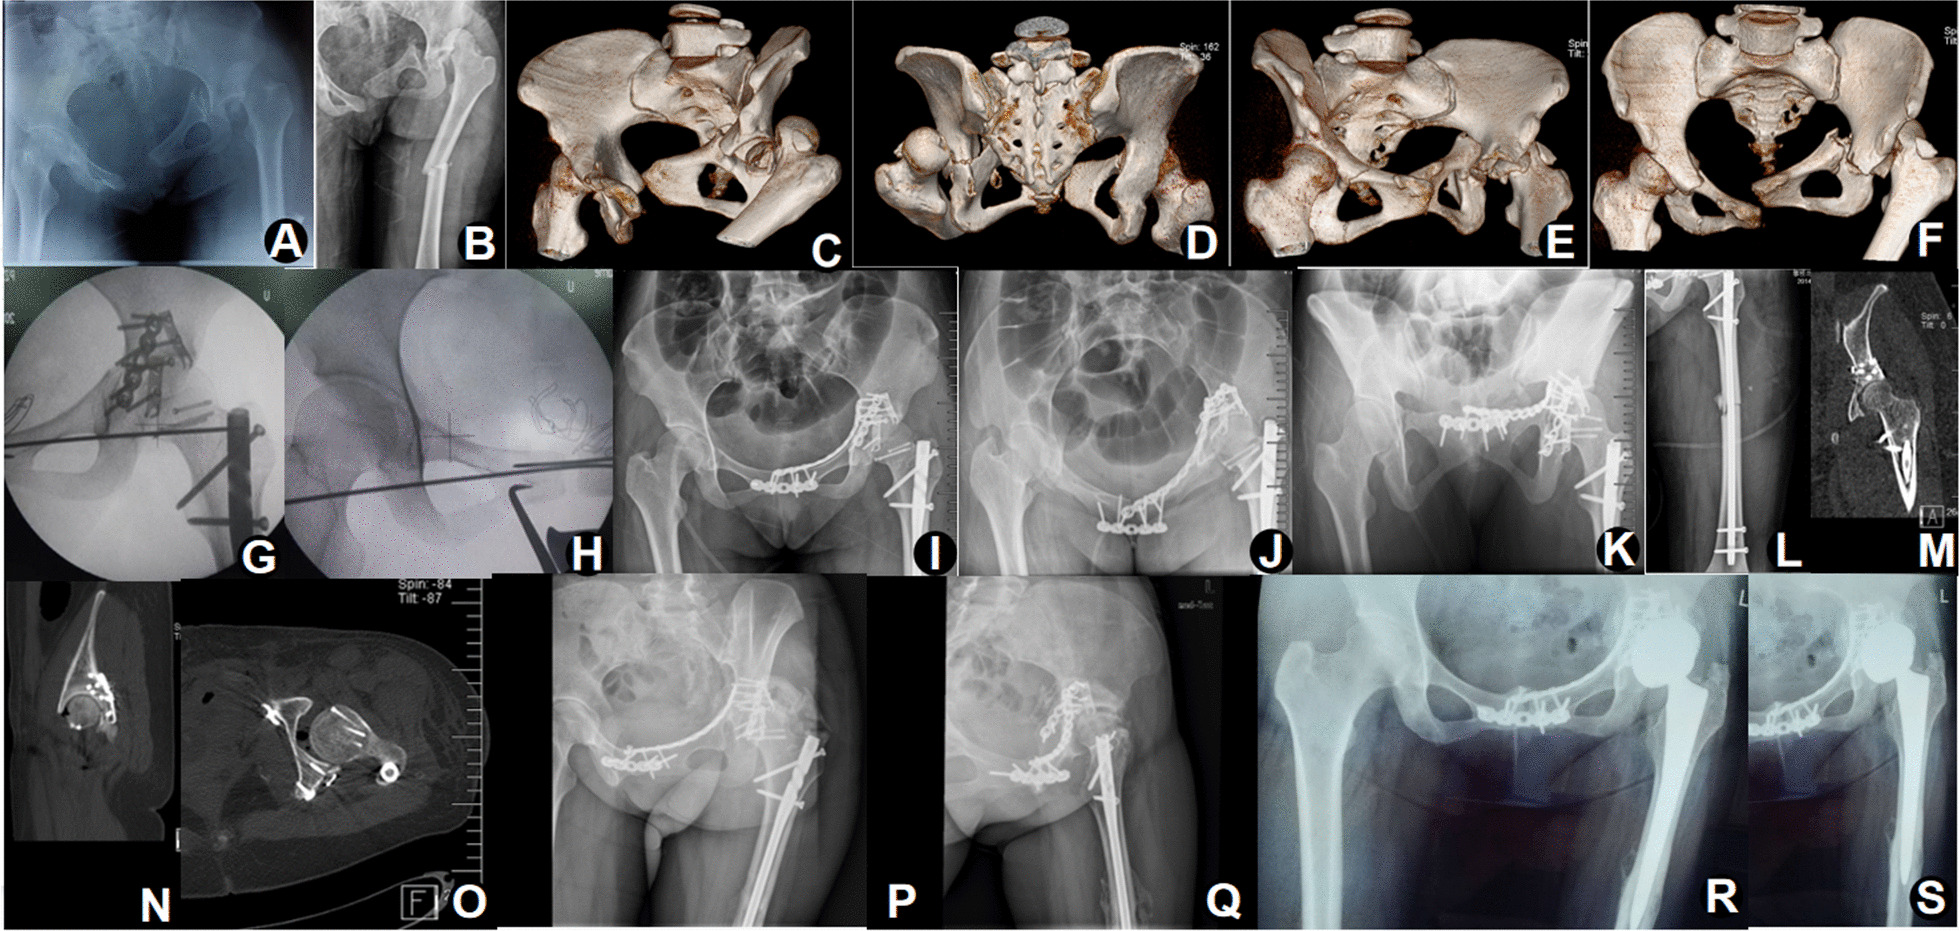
A 27-year-old female treated with injury control theory. Preoperative images suggested type C floating hip injury revealing a type C floating hip (A–F). An antegrade intramedullary fixation of femoral shaft fracture, followed by posterior fixation of the femoral head and posterior wall of the acetabulum and finally anterior fixation of the anterior ring and anterior column of the acetabulum on the seventh day after the injury (G, H). Immediate postoperative radiographs showing satisfactory reduction of all fractures (I–L). Postoperative CT suggesting anatomic reduction of acetabular fracture (M–O). Radiographs at 2 years of follow-up revealing severe post-traumatic osteoarthritis of the left hip with heterotopic ossification (P, Q), which was managed by implant removal and left THA at 3.5 years after the initial surgery (R), and the radiograph of 1 year after arthroplasty indicating no loosening of the prosthesis (S)
